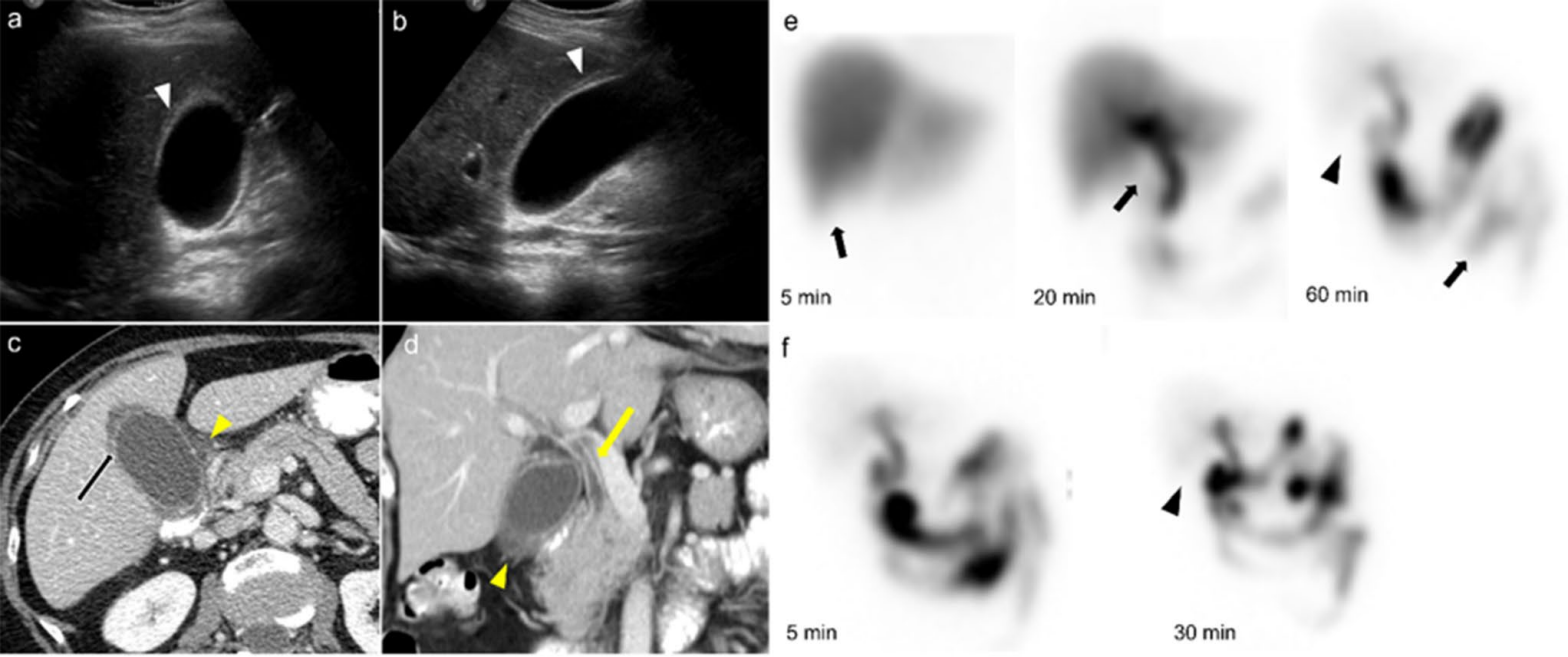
79-year-old female with right upper quadrant colicky postprandial pain. Ultrasound axial (image a) and long axis (image b) demonstrates wall thickening and hypoechogenicity in the wall due to edema (arrowheads). CT and scintigraphy scans were performed after the ultrasound. CT axial (image c) and coronal view (image d) demonstrates wall thickening (arrowhead), mucosal hyperenhancement (arrow), pericholecystic stranding (yellow arrowhead) and extrahepatic bile duct wall thickening and increased enhancement (yellow arrow) secondary to inflammation. The scintigraphy scan (image e) anterior image shows prompt uptake of the radiotracer by the liver (arrow), prompt excretion into the intrahepatic ducts, common bile duct by 20 min (arrow) and normal transit into the small bowel (arrow). There is no visualization of the gallbladder by 60 min (arrowhead). 2 mg Morphine was given intravenously. 30 min after the Morphine administration, there is no gallbladder visualization (arrowhead, f)
